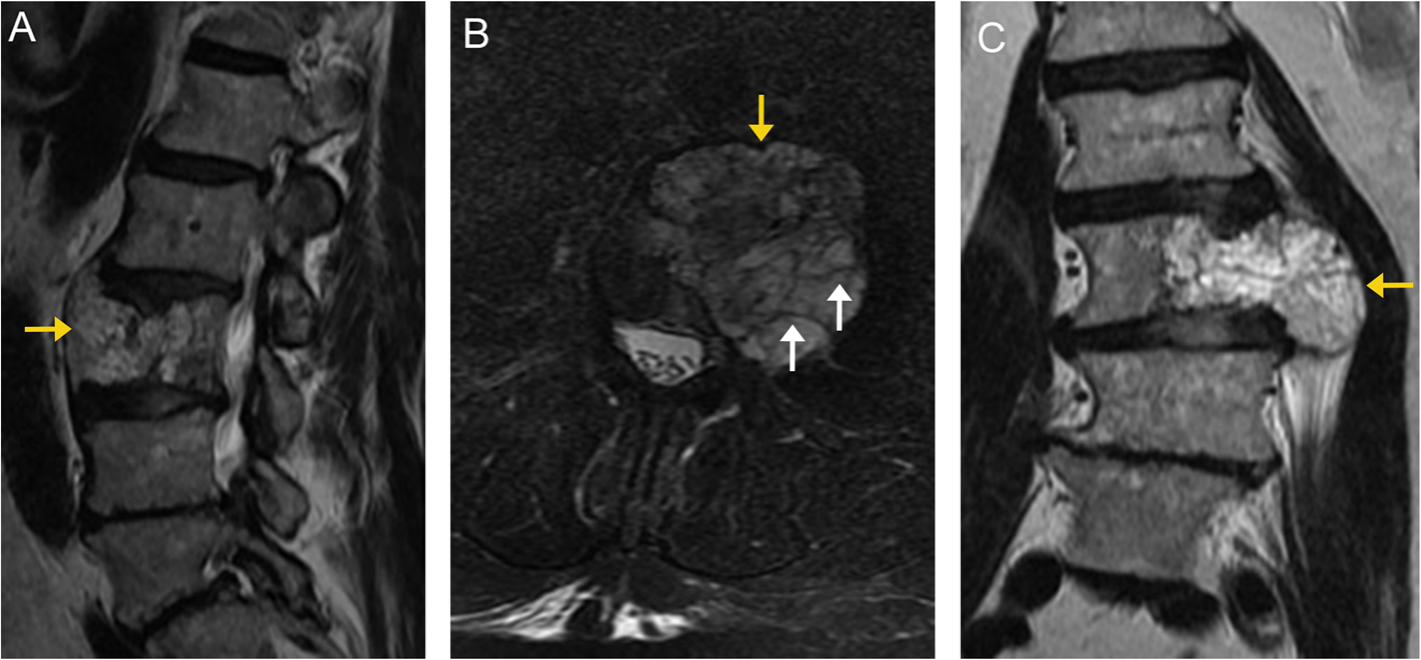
Chordoma. Sagittal T2-weighted (a), axial T2-weighted fat-saturated (b), and coronal T2-weighted (c) images demonstrate a heterogeneously hyperintense, expansile and destructive lesion (orange arrows) involving the L3 vertebral body. Numerous internal septa (white arrows) are seen within the lesion, creating a “honeycomb” configuration. The lesion extrudes out and over the left aspect of the veretebral body (creating a "mushroom" appearance), and displaces the adjacent paraspinal musculature
